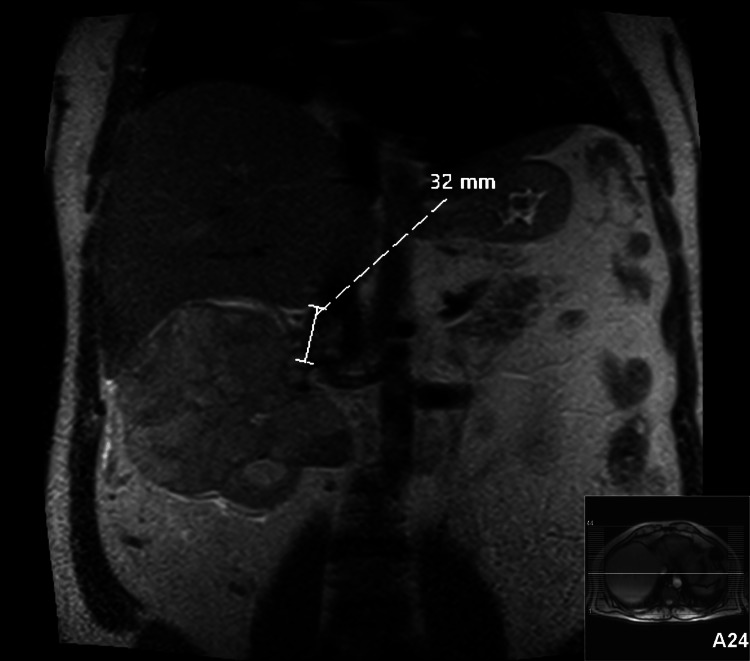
Abdominopelvic magnetic resonance imaging: a 32 mm tumor thrombus within the right renal vein, extending into the inferior vena cava
